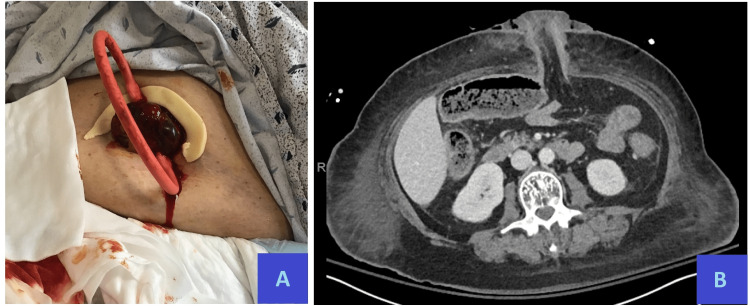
(A) Postoperative day three, loop colostomy with venous congestion and contact bleeding but normal appearance of peristomal skin. Additionally pictured, tan-colored skin barrier ring and red rubber catheter stoma bar. (B) Postoperative day four, CT scan showing left upper quadrant loop colostomy with non-specific subcutaneous edema. CT: computed tomography
